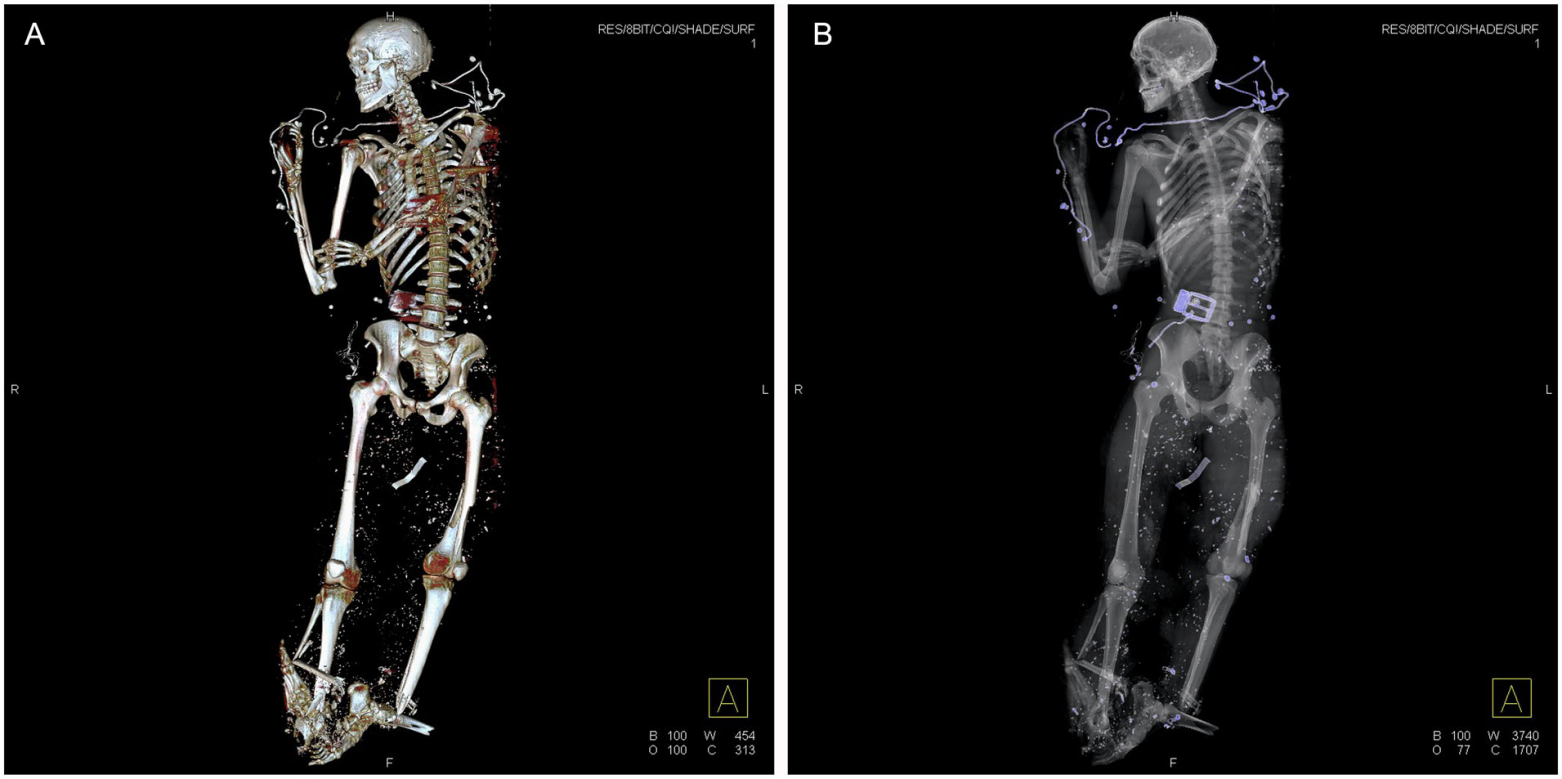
Postmortem CT-scan of victim: penetrated foreign objects (shrapnel) and partial avulsion underlegs. (A) Three-dimensional image of total skeleton. (B) Conventional radiologic overview of total body.
